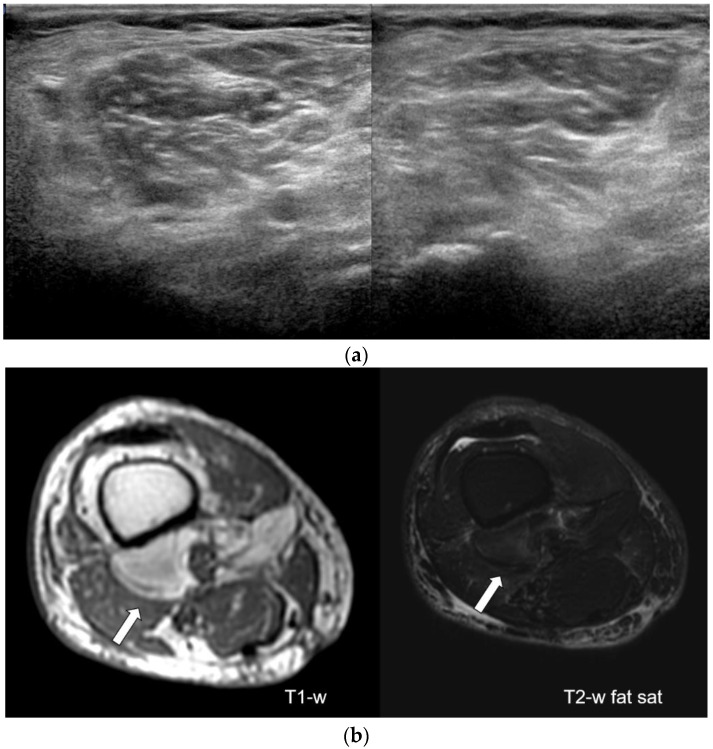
A 78-year-old man operated for a localized liposarcoma of the popliteal fossa in follow-up ultrasound images (a) obtained at the popliteal fossa were reported as negative for local recurrence with no recurrence demonstrated in the follow-up. MRI images (b) show a deep-located (white arrow) nodular lesion with MRI signal consistent with fat on T1-weighted sequences and T2-weighted sequences with fat saturation. A slight hyperintensity on T2-weighted sequences with fat saturation was present. In this case, ultrasound was reported negative for recurrence, whereas MRI was reported positive.
